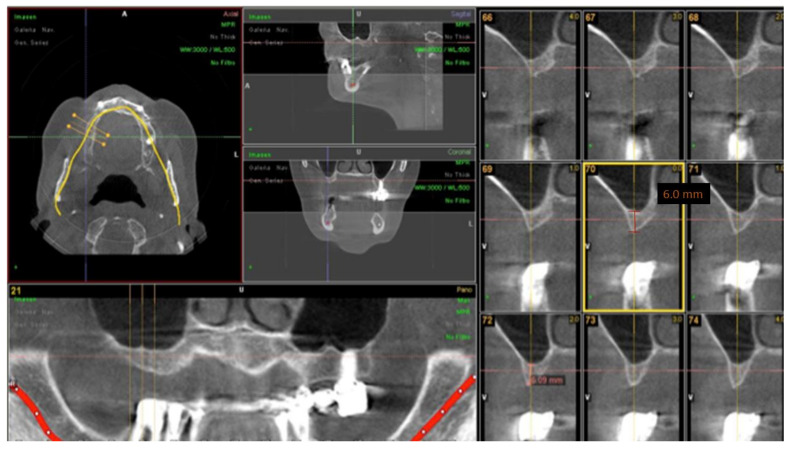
CBCT prior to maxillary sinus elevation surgery.
